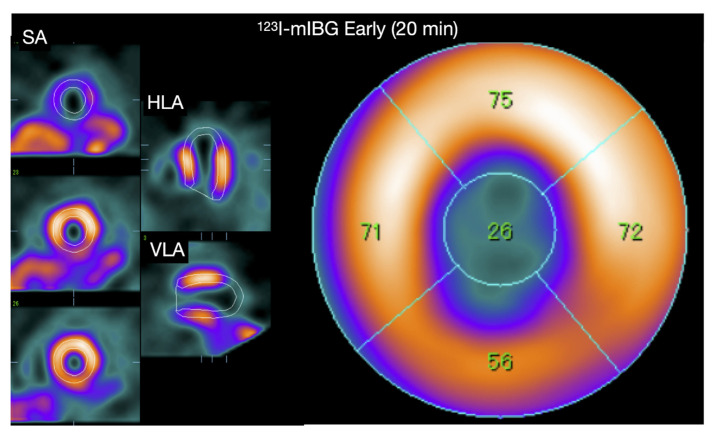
A 60-year-old Japanese woman diagnosed with Takotsubo syndrome. The ^123^I-*m*IBG early SPECT show a clear defect in the apical region, and the late image also showed a similar defect (figure not shown). The early and late planar H/M ratio of this patient are shown in Figure 2, showing preserved ^123^I-*m*IBG uptake globally despite the severe apical defect.
